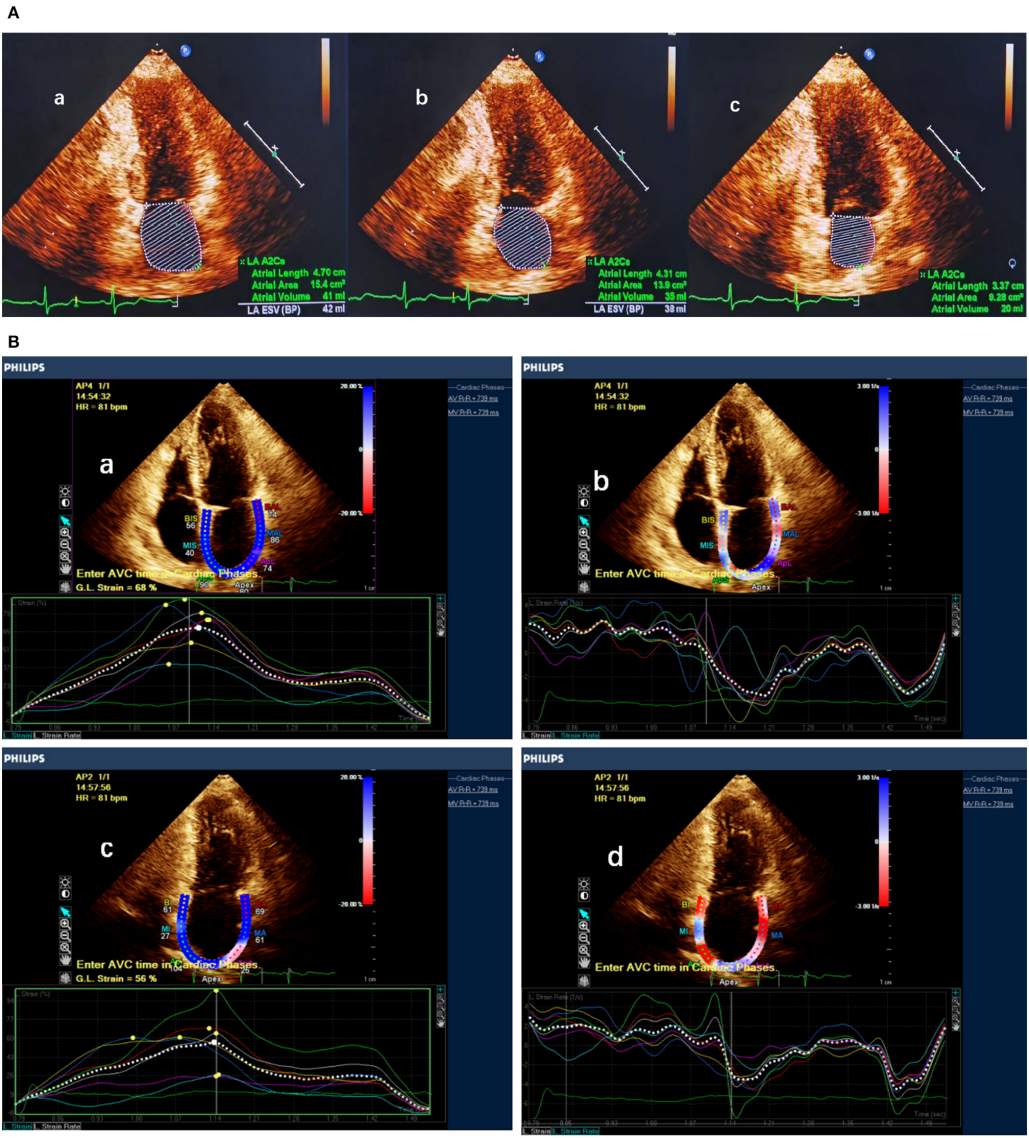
LA function measured by LA volume and speckle tracking echocardiography. (A) Measurement of maximum LA volume, before atrial contraction LA volume and minimum LA volume. (B) Measurement of LA strain and strain rate in apical two- and four-chamber view. a,b Atrial strain and strain rate measured in apical four-chamber view. c,d Atrial strain and strain rate measured in apical two-chamber view.
